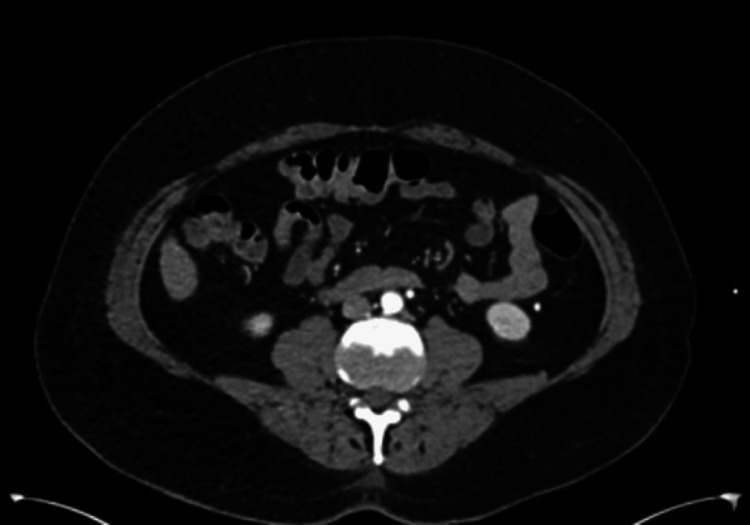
CT angiogram showed intact abdominal aorta.
